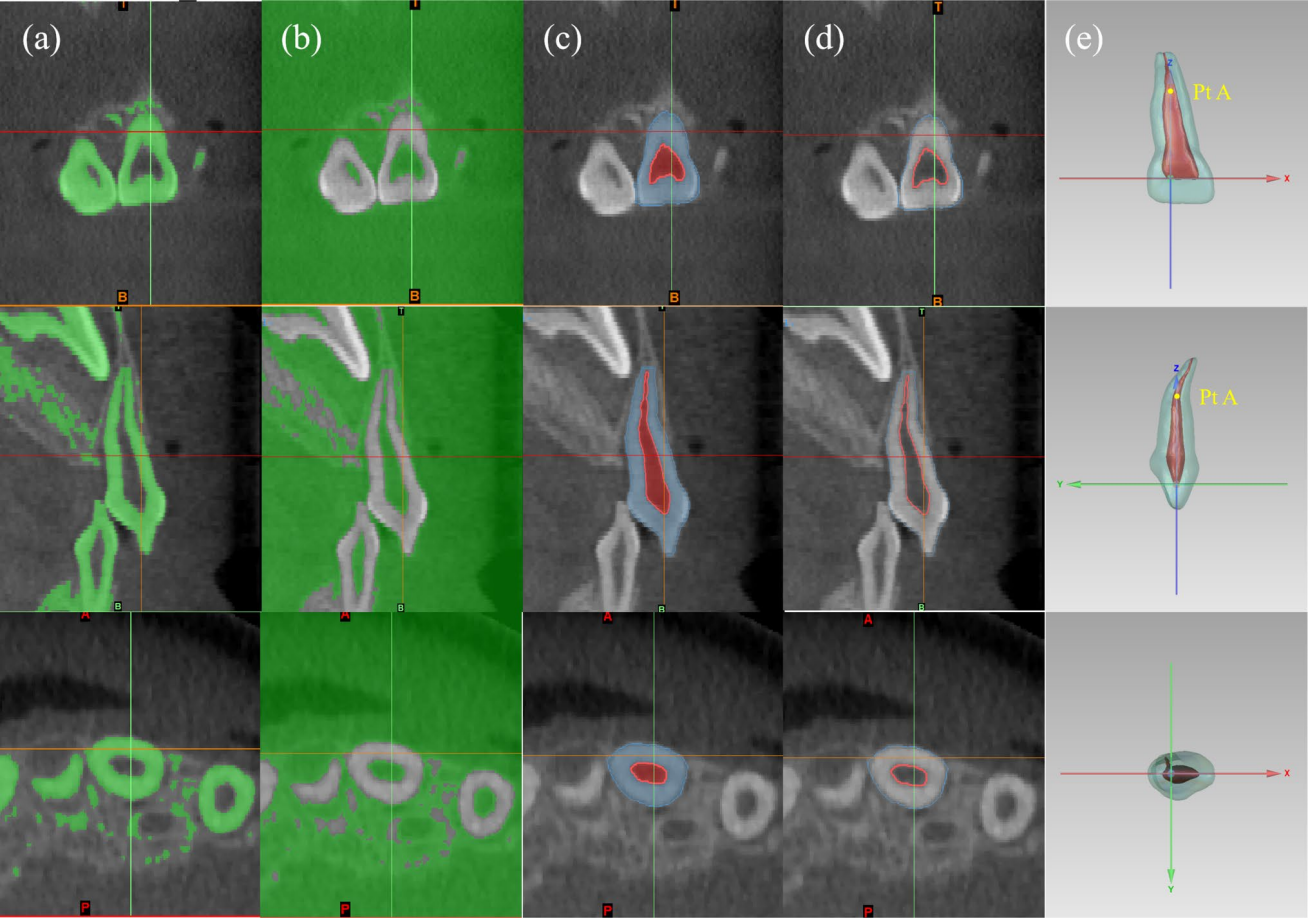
Segmentation and coordinate adjustment. a Threshold selection for hard tissue; b threshold selection for pulp chamber and canal; c extracted hard tissue (blue) and pulp chamber and canals (red); d profile lines for hard tissue (blue) and pulp chamber and canals (red), consistent with the original cone-beam computed tomography (CBCT) scan; e coordinate axes adjustment. Point A indicates the midpoint of the section at the apical labial curvature
